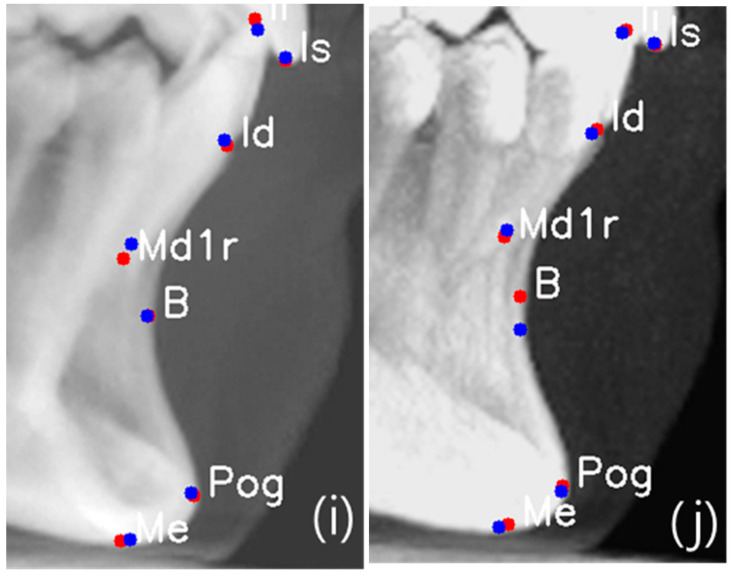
Comparison of AI prediction between CBCT-LC (a,c,e,g,i) and MIP-LC (b,d,f,h,j); Orbitale c and d; Maxilla e and f; Porion g and h; and Mandible i and j. The red dot is manual identification (truth ground), and the blue dot is AI prediction.
